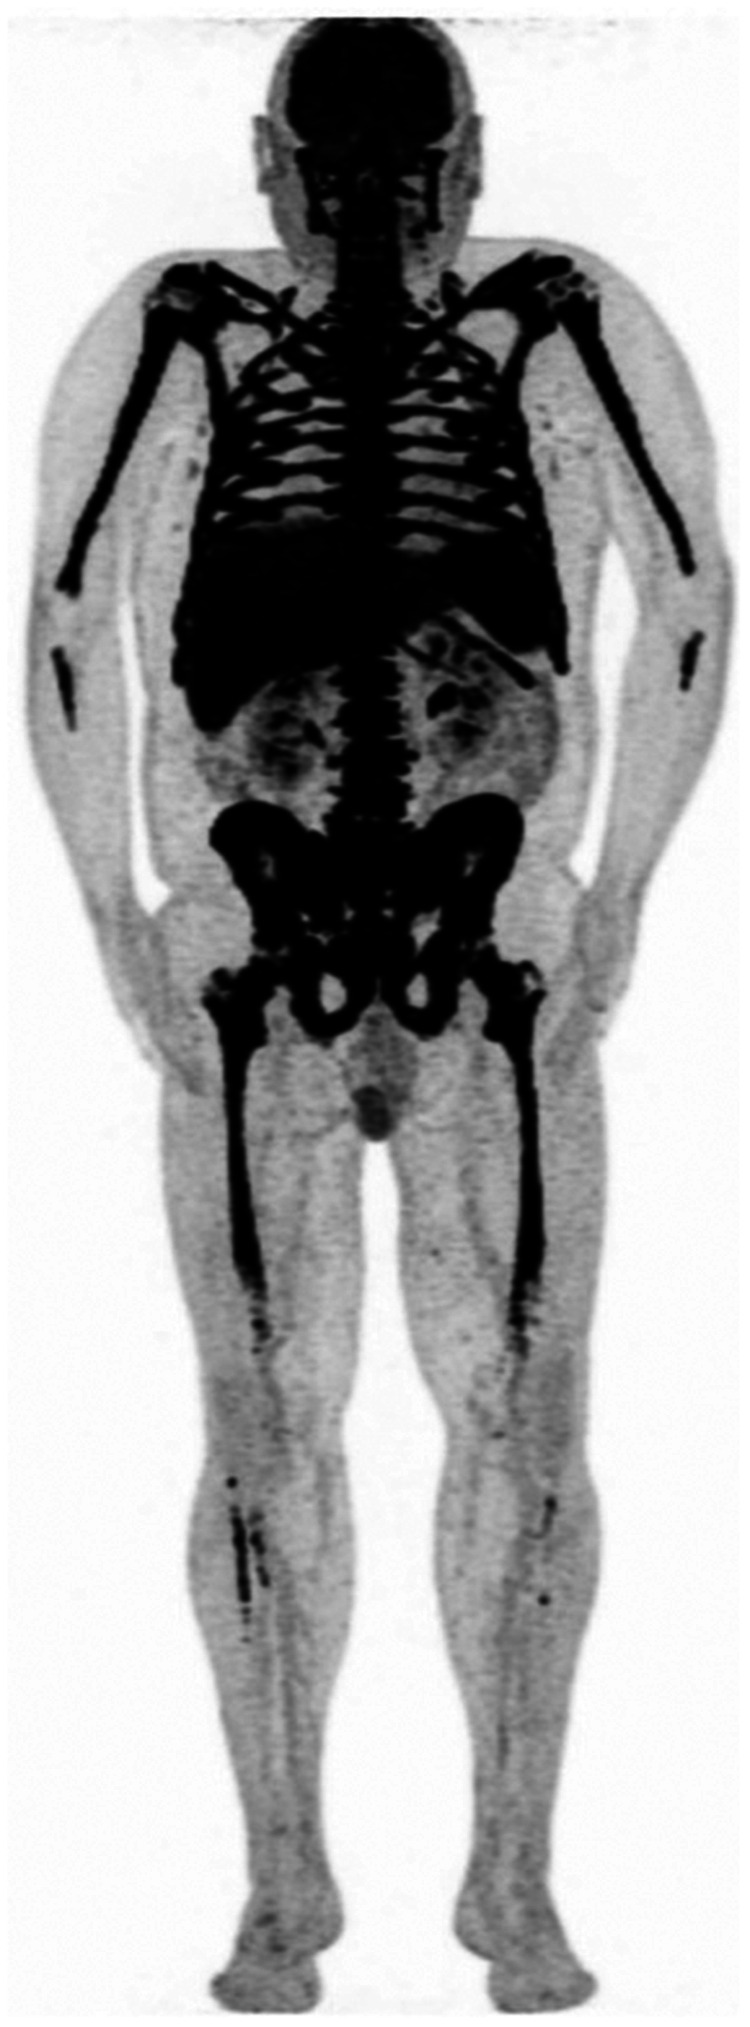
^18^F-FDG PET maximum-intensity projection showing diffuse bone marrow involvement.
